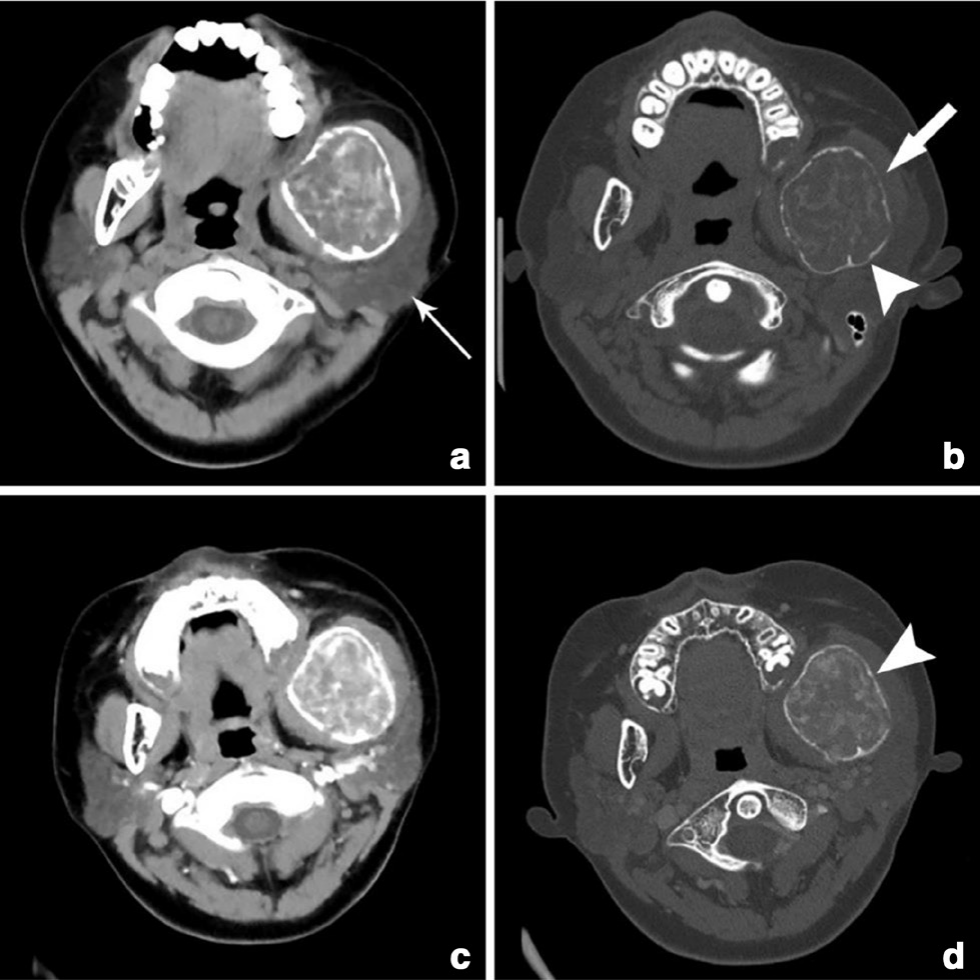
(a–d) Case 1: axial CT images without contrast (a, b) and with contrast (c, d) demonstrating expansile bony lesion of left mandible. The mass is well-circumscribed, radiolucent, cortical destruction and thinning (arrow head), granular bone pattern laterally (thick arrow). The adjacent soft tissue and masseter muscles are compressed and swollen (thin arrow).
